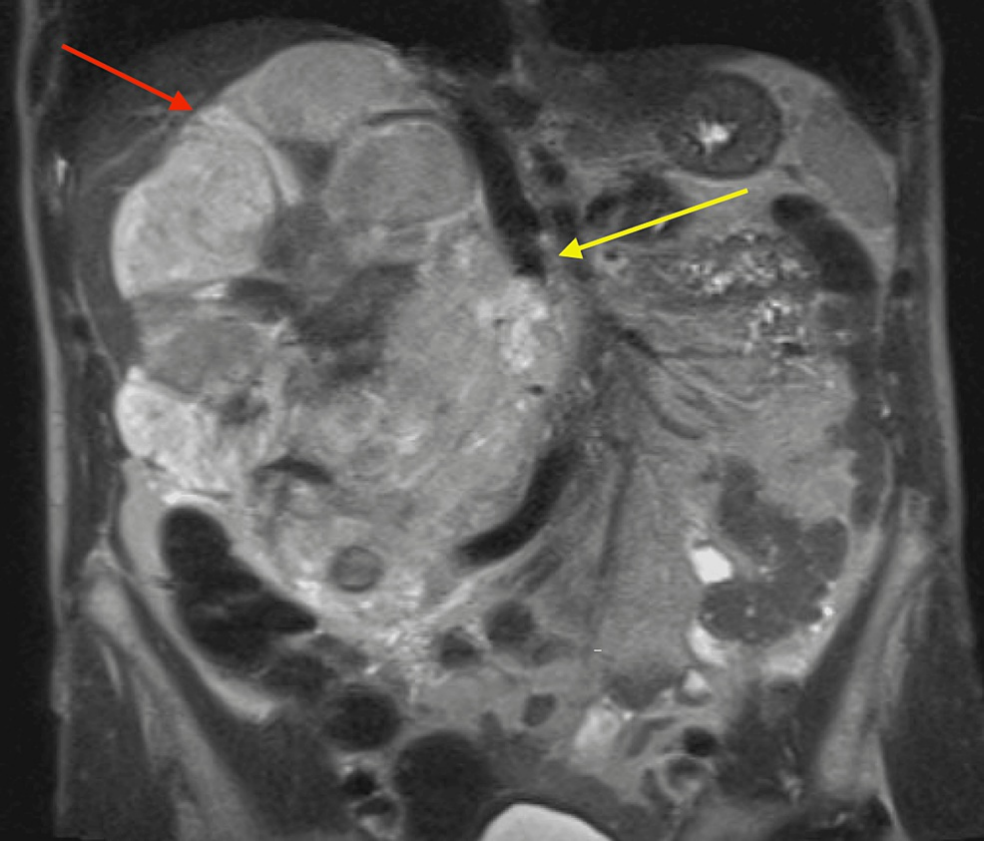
MRI adrenals with and without contrast coronal view revealing a large, T2 hyperintense heterogeneously enhancing mass (red arrow) in the right hemiabdomen, extending from right hemidiaphragm to right upper pelvis. Mass is seen compressing the inferior vena cava (yellow arrow).
